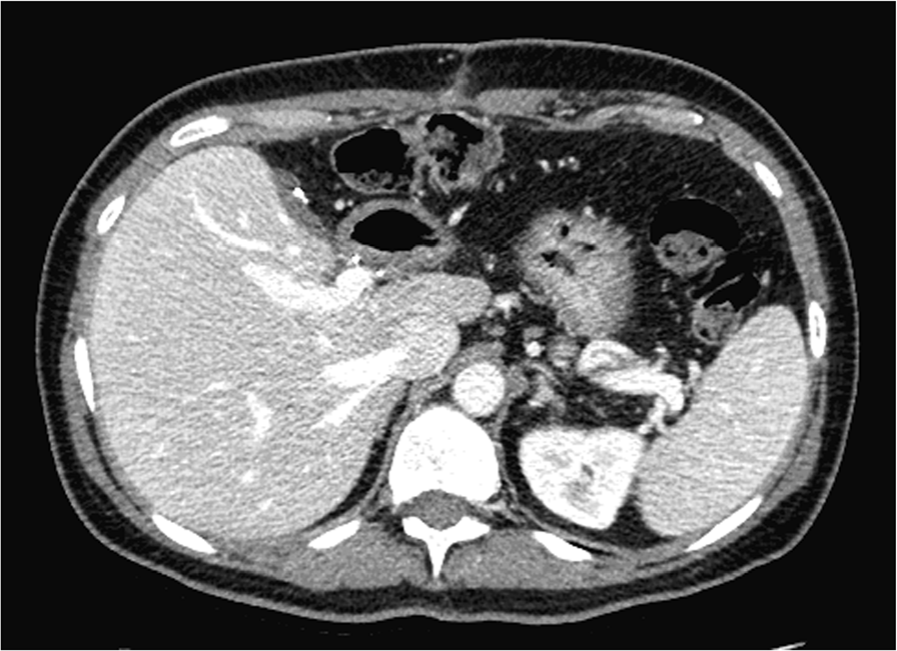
CT scan of the abdomen performed three months after resection of the left lobe of the liver demonstrating normal-appearing right lobe of the liver without evidence of new or recurrent metastatic disease.
